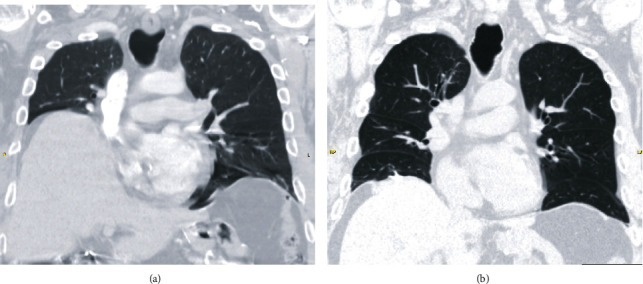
Coronal reconstruction of chest computed tomography (a) pre- and (b) postdiaphragmatic plication with noted improved lung expansion.
